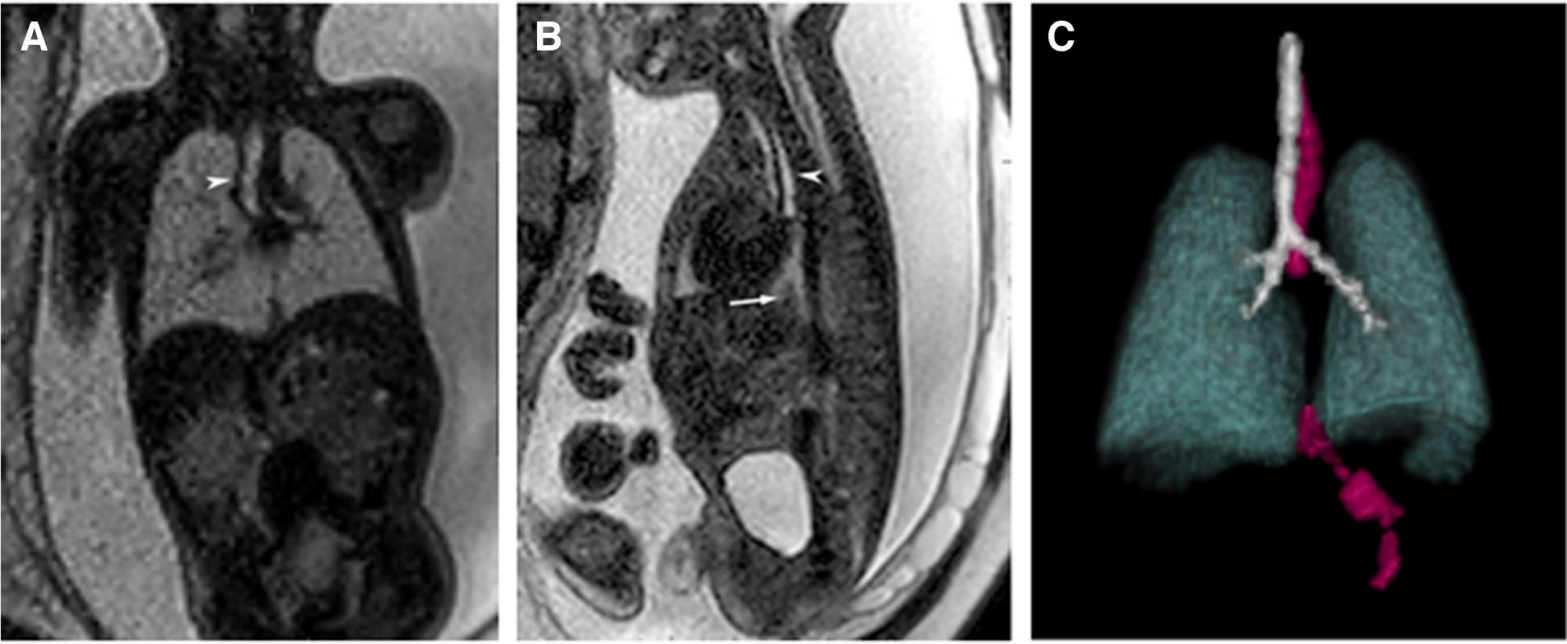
A Coronal and (B) sagittal T2-weighted SS-FSE fetal MRI images of a fetus with suspected esophageal atresia scanned at 33 2/7 weeks, showing a dilated upper esophageal pouch (*white arrowheads* on A and B). A large gap is seen between the atretic proximal esophagus and a small remnant of the distal esophagus (*white arrow* in B). C 3D-rendered images of the lungs (*green*), trachea and bronchi (*white*), and esophagus (*magenta*) show the atretic esophagus ending just below the carina and a large gap between the atretic upper esophagus and the distal gastroesophageal junction
